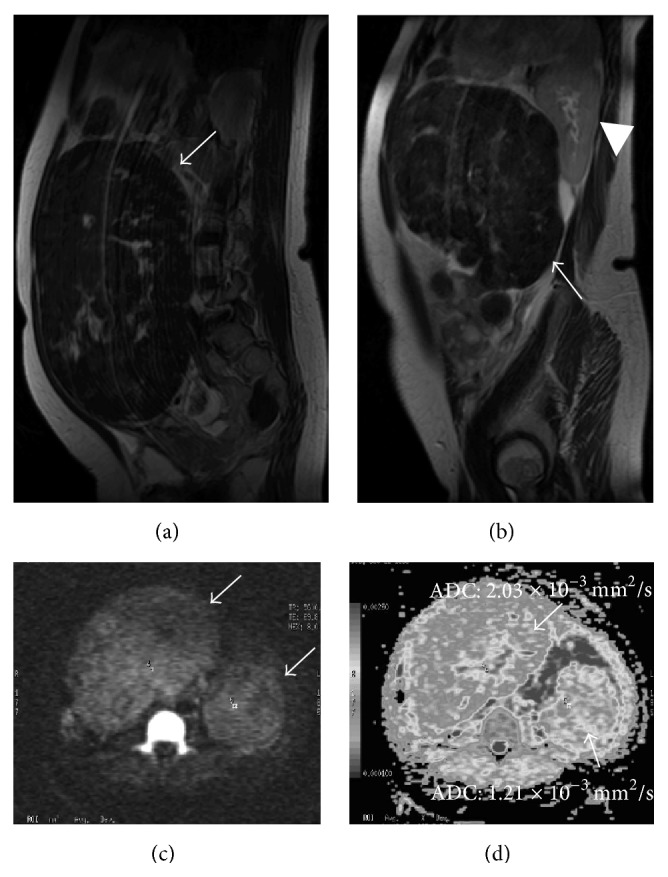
Two large tumors ((a–d), arrow) reached kidney ((b), arrow head). These tumors showed low intensity on T2-weighted image ((a) and (b), arrow) and intermediate intensity on diffusion-weighted image ((c), arrow). The apparent diffusion value was 2.03, 1.21 × 10^−3^ mm^2^/s ((d), arrow); thus, we did not consider that these tumors were as leiomyosarcoma.
